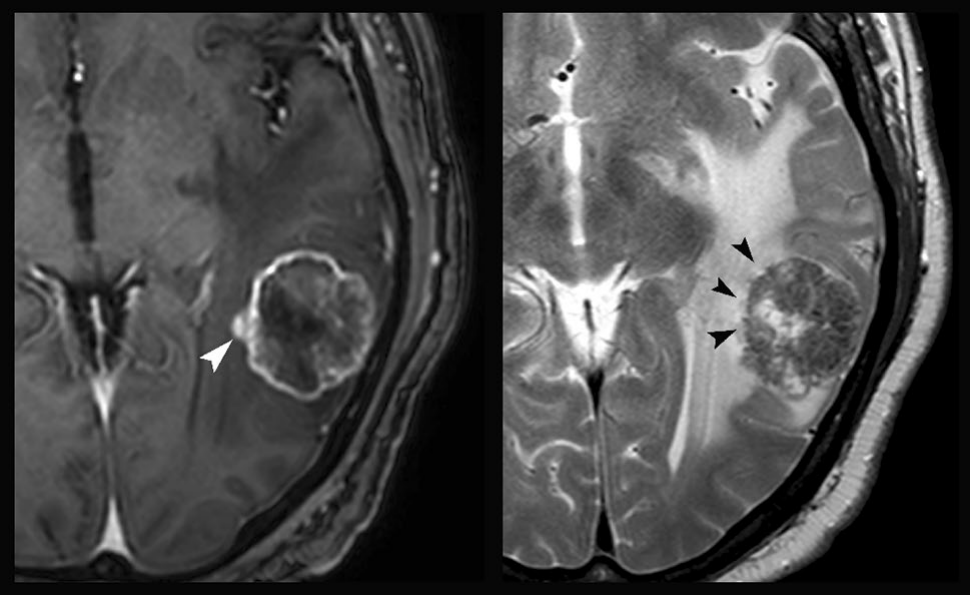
ROI positioning on the images of a patient affected by SBM (same patient as in images a and c from Fig. 1): post-contrast T1-weighted (left) and axial T2-weighted (right) images. The white arrowhead indicates the ROI positioned in the enhancing region. The three black arrowheads indicate the ROIs positioned in the peri-enhancing lesion within 1 cm from the enhancing lesion. A fifth ROI (not shown) was positioned in the white matter of the contralateral hemisphere for normalization purposes
